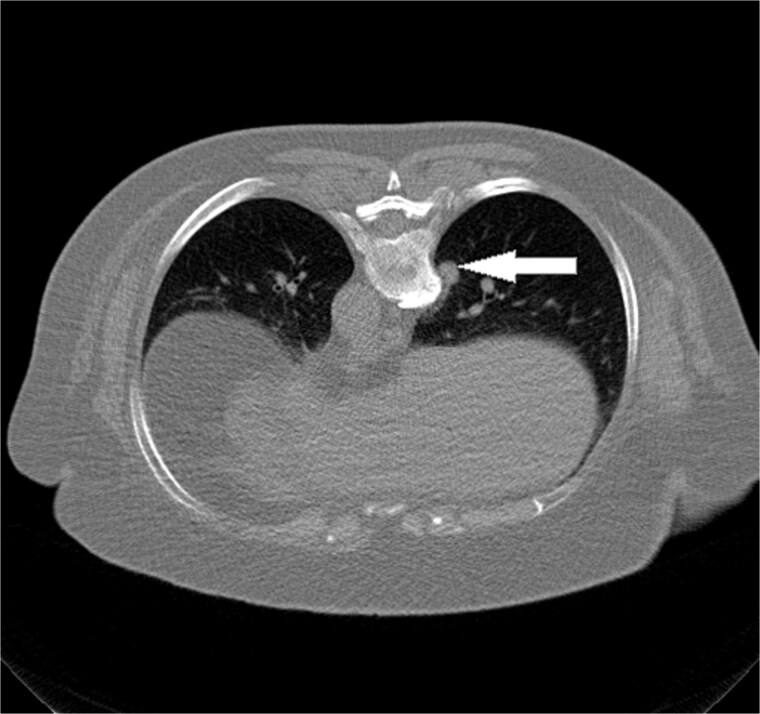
Axial non-contrast chest CT obtained prior to ablation demonstrates a solitary, well-circumscribed pulmonary nodule in the right lower lobe abutting the pleural surface (arrow). The lesion measured 11 mm in maximal diameter and was suspicious for breast cancer metastases.
